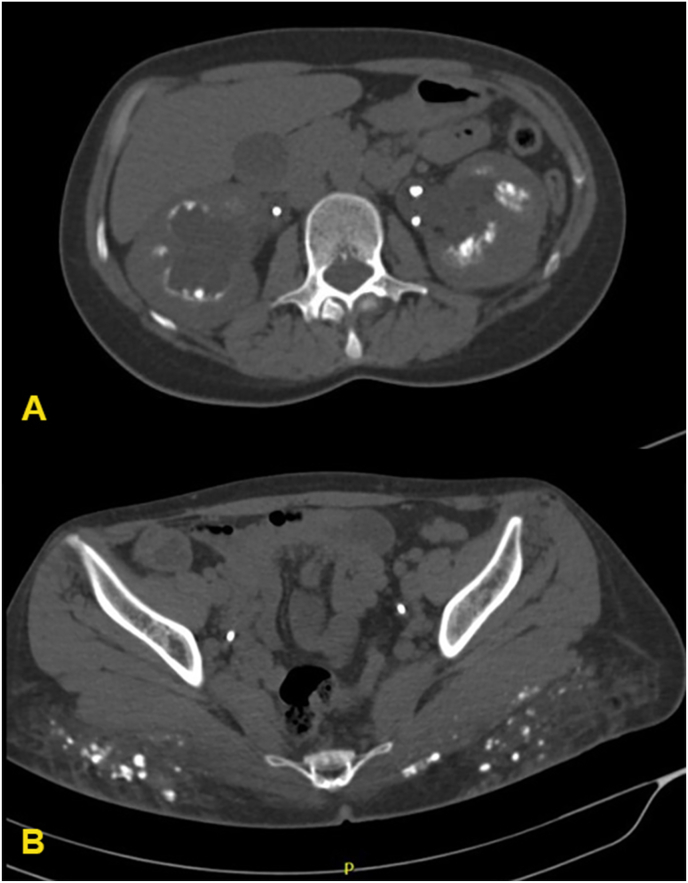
CT scan of the abdomen showing (A) bilateral nephrocalcinosis and (B) innumerable small calcifications with surrounding fat stranding in the gluteal muscles and the overlying fat plane.
